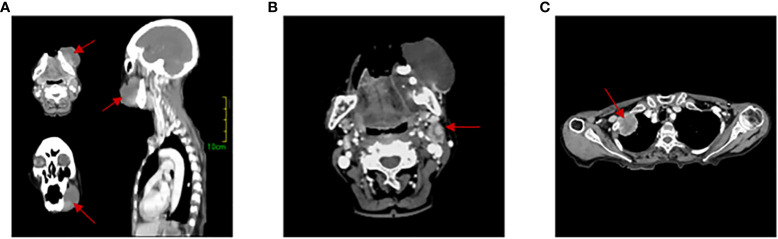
Computed tomography (CT) scans. (A) 3D image of gingival tumor. (B) Lymph node metastasis area. (C) Lung metastases lesion. The red arrow indicates the location of the lesion.
